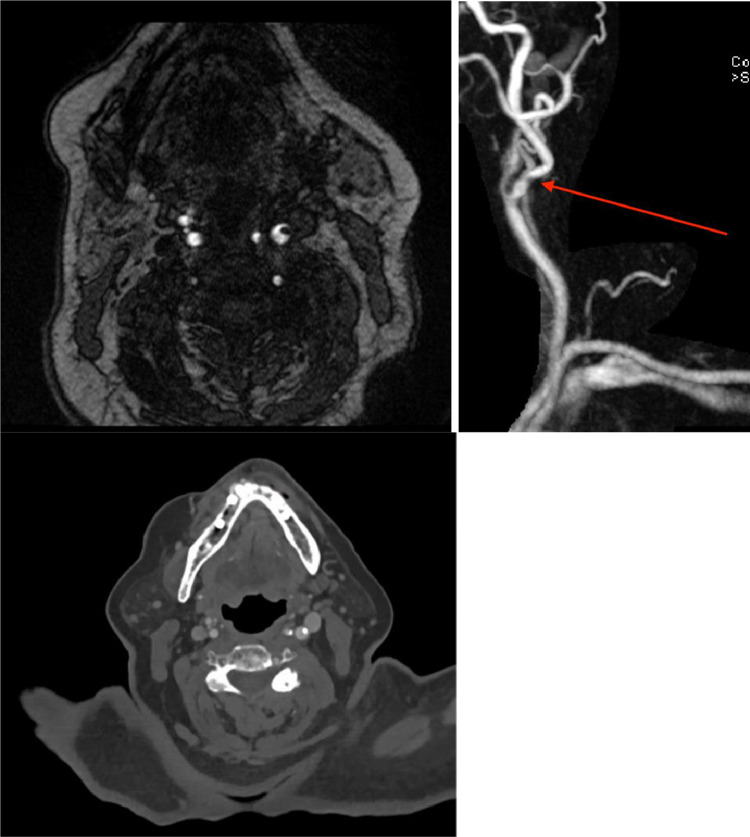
As pictured above: 9.5 h after presentation, MRA Neck demonstrating a lower filling defect in the left carotid lumen, likely present (left), but not as well-seen on the post-contrast MRA imaging of the left internal carotid artery (middle) with the CTA (right) also matching the location of the plaque.
